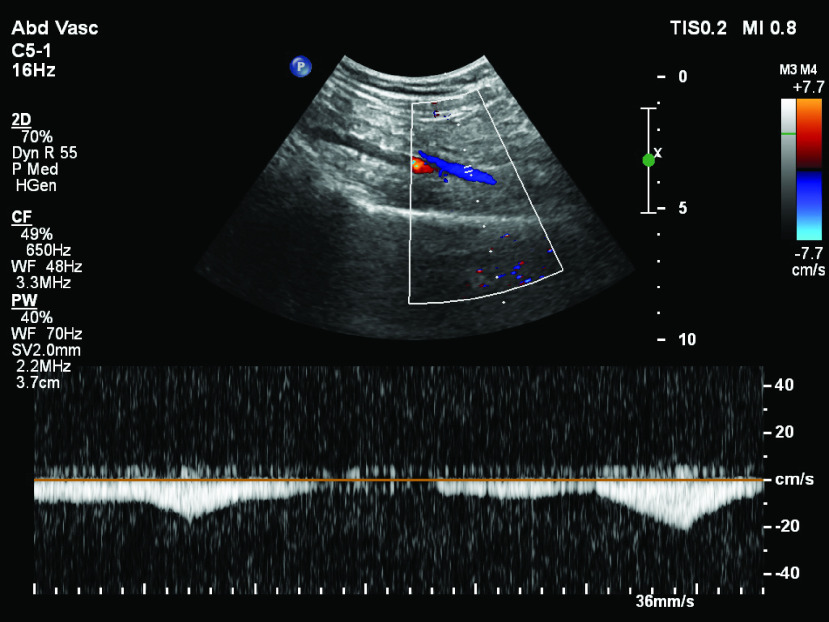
An image acquired from ultrasonography device while scanning the popliteal fossa region of the subject.
